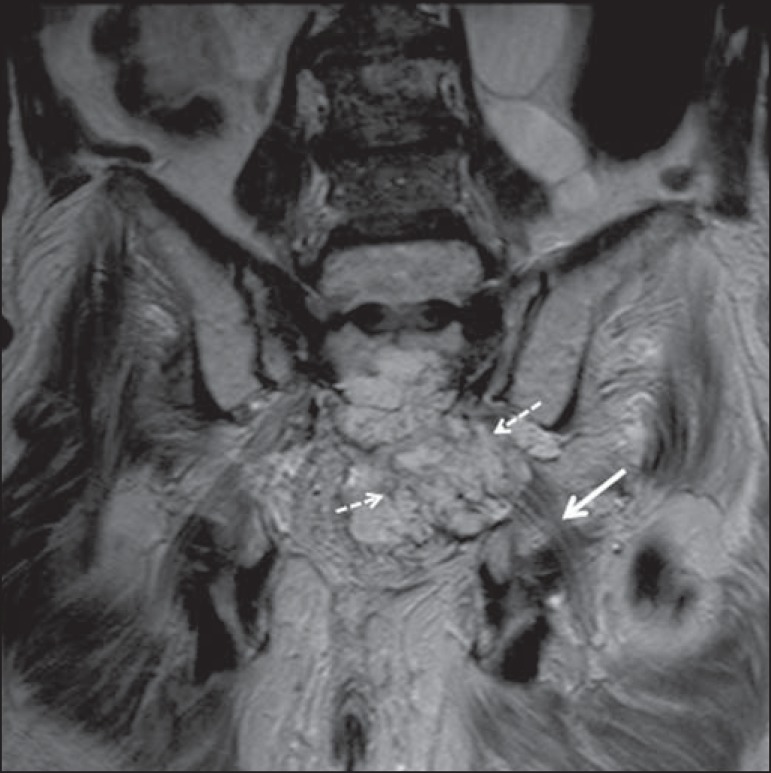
66-year-old male patient with rectal adenocarcinoma. Coronal T2-weighted
